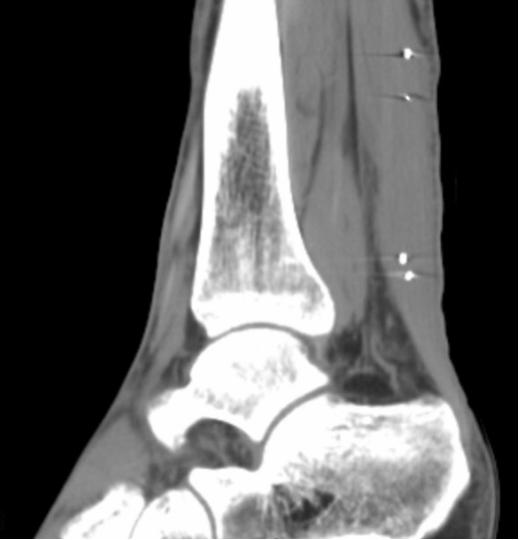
CT. CT after 12 weeks. Note the thick tendon callus. The two tantalum markers on each side of the healing rupture are enlarged due to artefacts.
